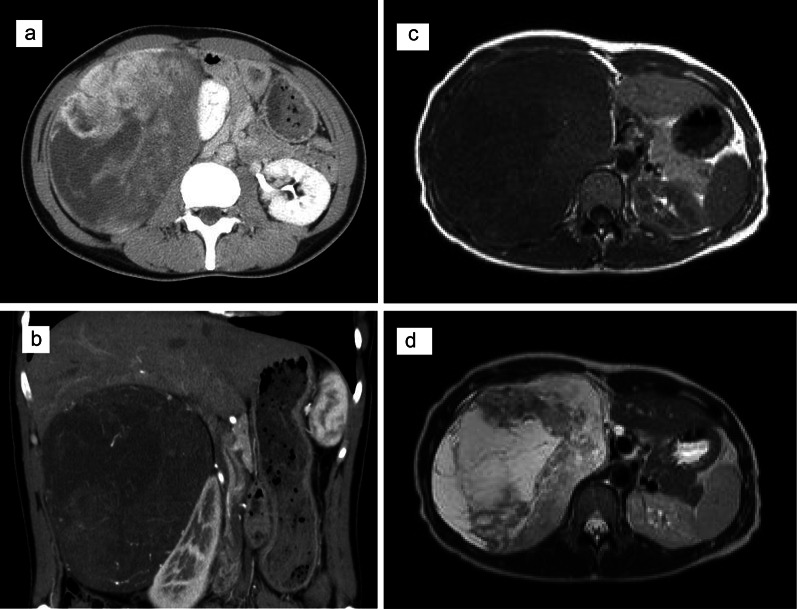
CT and MRI imaging before treatment of primary tumor. a Abdominal CT shows a solid encapsulated mass with nonuniform internal structure approximately 16 × 16 × 10 cm, compressing the right kidney and duodenum to the caudal side; b contrast-enhanced CT coronary cut; c MRI shows association-clear, T1-weighted tumors with predominantly low to equal signals; d T2-weighted image showed a high signal intensity tumor with low signal intensity area inside
